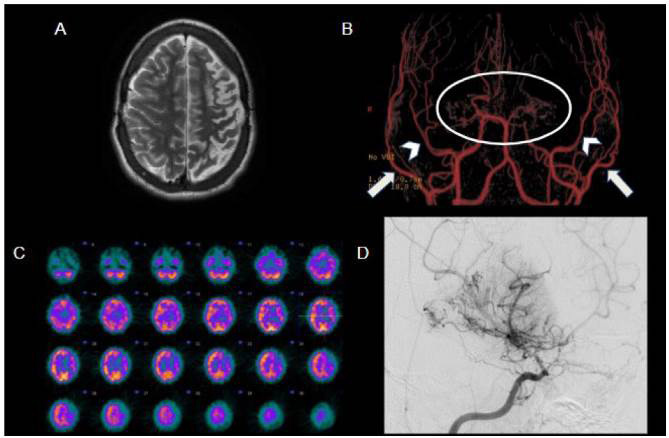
Moyamoya syndrome secondary to sickle cell disease. 18-year-old male patient with sickle cell disease and history of multiple cerebrovascular events, who underwent bilateral encephalo-duro-arterial synangiosis (EDAS). Axial T2WI (A) shows a large left frontoparietal area of encephalomalacia. MRA (B) depicts progressive tapering of the distal internal carotid arteries, culminating in distal subocclusion/occlusion (circle), with extensive collateral vessel network deriving from external carotid arteries; the dilated branches of these arteries, especially the medial meningeal arteries (arrowheads) and superficial temporal arteries (arrows), are related to EDAS. SPECT (C) demonstrates reduced perfusion in the left cerebral hemisphere, while the right hemisphere is relatively preserved, possibly due to EDAS. Digital subtraction angiography (D) on another sickle cell patient (13 years old) outlines the classical angiographic pattern of moyamoya syndrome (internal carotid artery stenosis with compensatory hypertrophy of lenticulostriate arteries).
